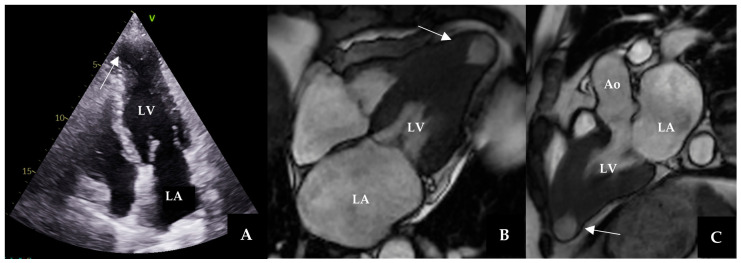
Apical aneurysm (arrow) in a 2−chamber view by TTE (A) and in 4−chamber (B) and 3−chamber (C) views by CMR. Ao: Aorta; CMR: Cardiac magnetic resonance; LA: Left atrium; LV: Left ventricle; TTE: Transthoracic echocardiography.
